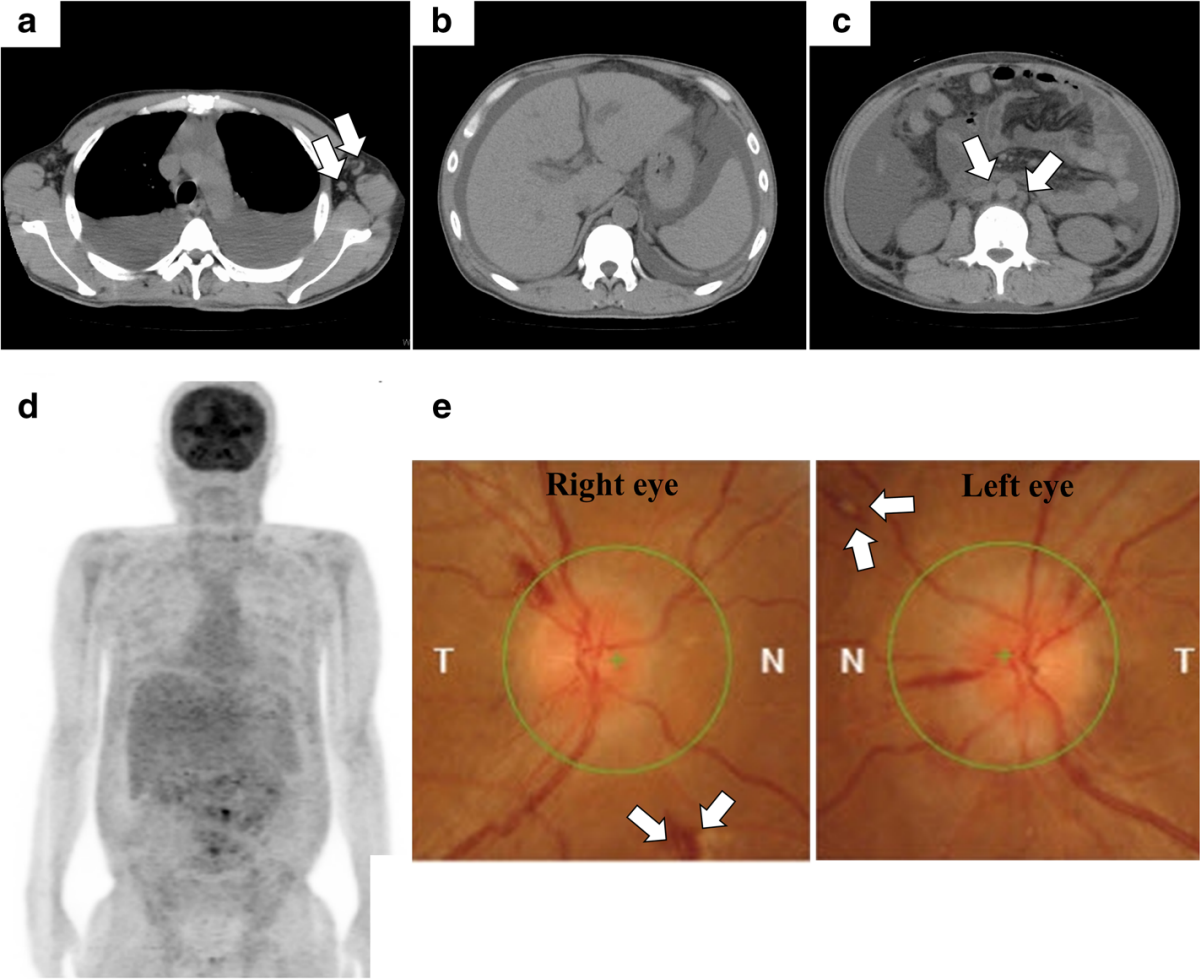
Imaging findings. a–c Computed tomography images on the day of admission. a Massive pleural effusion and slightly enlarged axillary lymph nodes are observed (arrows). b Hepatosplenomegaly is seen. c Massive ascites and slightly enlarged para-aortic lymph nodes are observed (arrows). d
